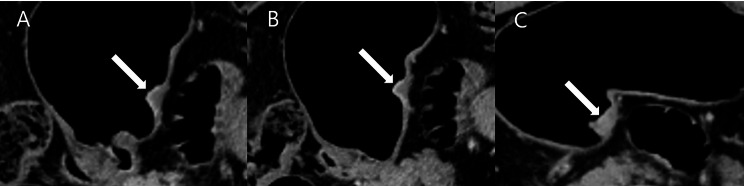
A patient with differentiated T1b sm2/3 stomach cancer. A and B. In the arterial and portal phases, the lesion (arrow) shows wall thickening characterized by double-layered enhancement, comprising an inner enhancing layer and an outer non-enhancing layer. Both phases demonstrate an outer layer with a thin enhancement pattern resembling the normal gastric outer layer, indicative of a negative CT transmural sign. C. In the delayed phase, the thickened gastric wall exhibits transmural enhancement as a single layer (arrow), representing a type I positive dynamic CTTM sign. The CTTM pattern is classified as 0-0-1
